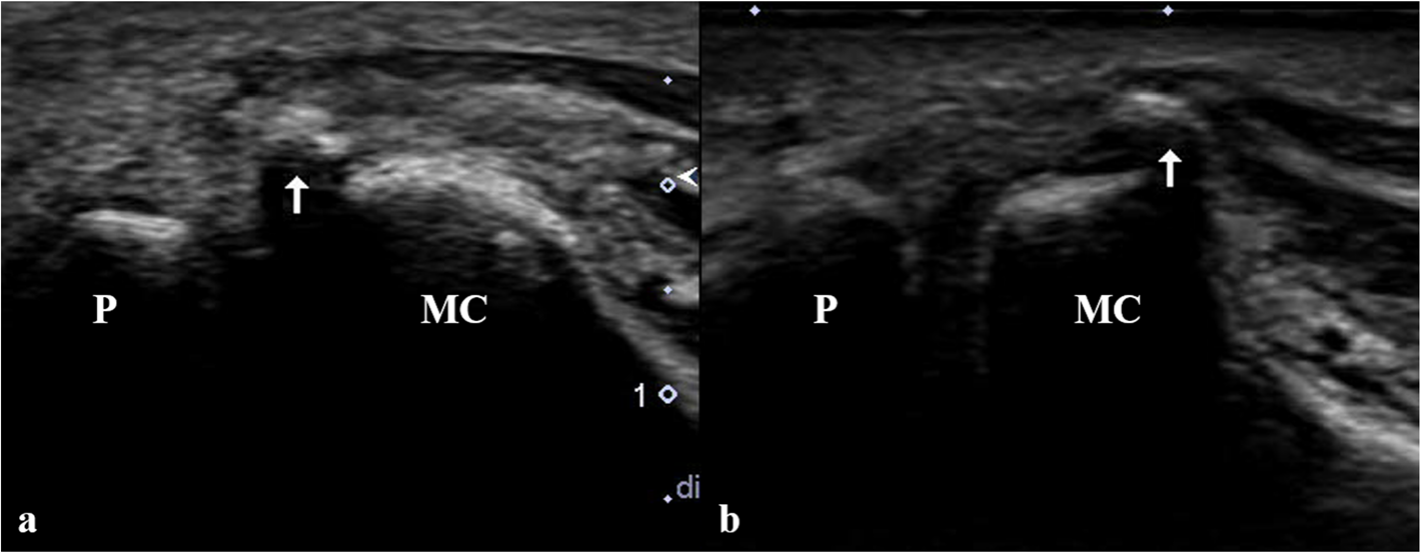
Longitudinal scan of ultrasound on volar surface of the 1st metacarpophalangeal joint shows distal displacement of the radial sesamoid bone (arrow) into the joint space (a). After reduction, the radial sesamoid has relocated to the radial condyle of the metacarpal head (b). P: proximal phalangeal base, MC: metacarpal head
